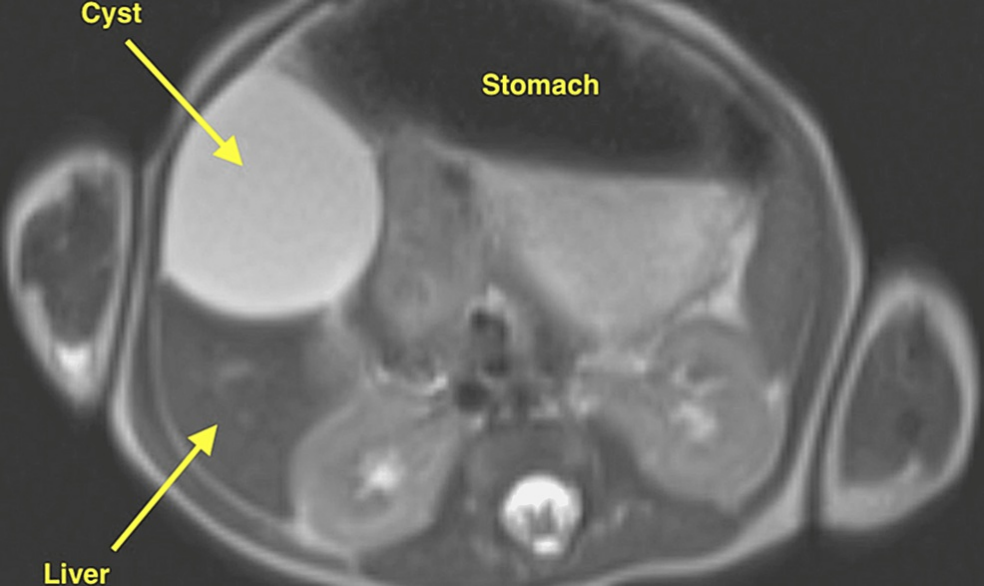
T2-weighted abdominal MRI for Case 2 The hyperintense cystic lesion was measured to be 3.5 x 2.1 x 3.7 cm.
